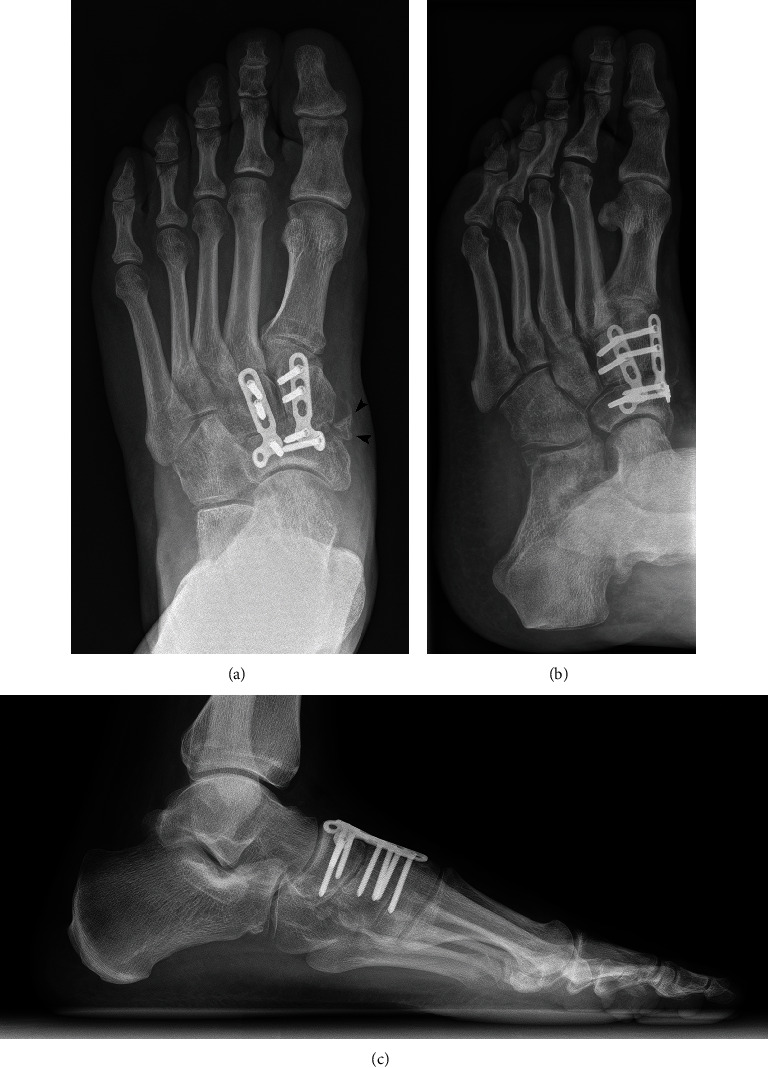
(a) Anteroposterior weight-bearing, (b) oblique, and (c) lateral weight-bearing X-rays of the left foot 10 months after the surgery. Black arrowheads show the osseous fragment next to the N-C1 joint responsible for the discomfort at the medial side of the midfoot.
